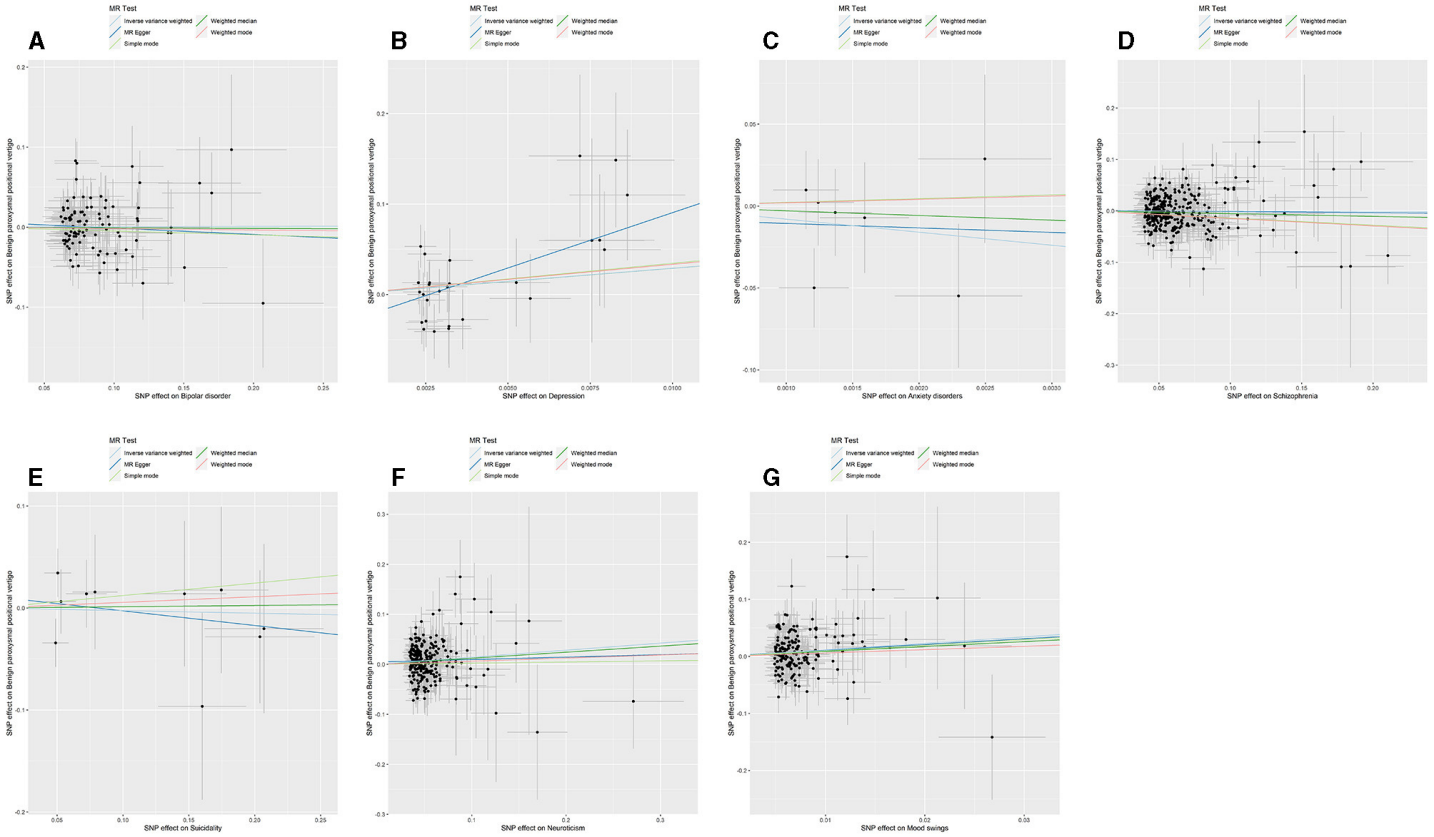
Summary view of the MR images derived from the IVW, MR–Egger, simple mode, weighted median, and weighted mode methods. (MR-PRESSO adjustment) (A) The MR analysis results for bipolar disorder and BPPV. (B) The MR analysis results for depression and BPPV. (C) The MR analysis results for anxiety disorders and BPPV. (D) The MR analysis results for schizophrenia and BPPV. (E) The MR analysis results for suicidality and BPPV. (F) The MR analysis results for neuroticism and BPPV. (G) The MR analysis results for mood swings and BPPV.
